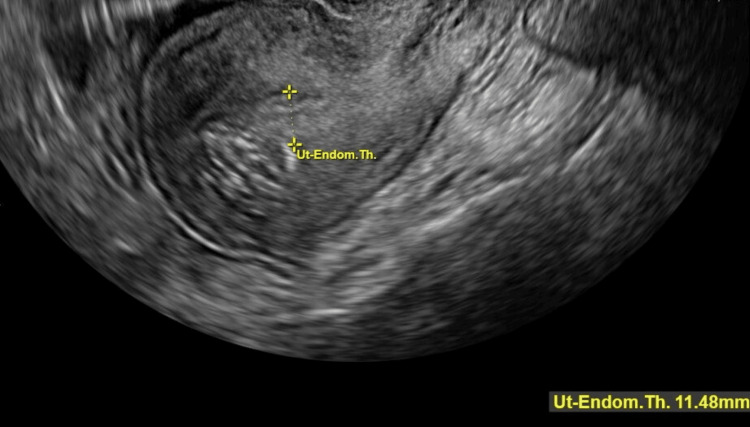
Ultrasound image showing the endometrial stripe measuring 11.48 mm
